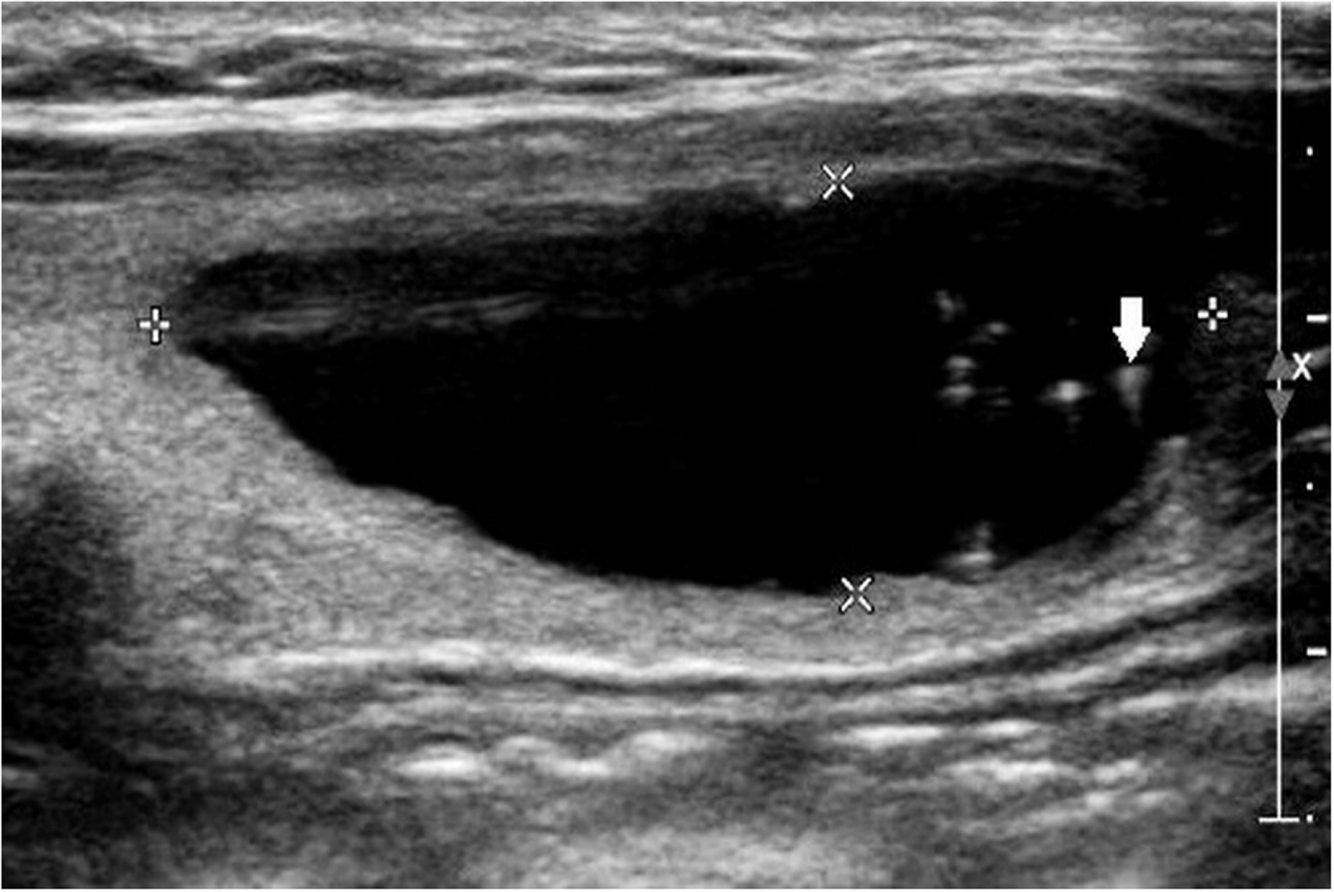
A 46-year-old woman with degenerated multinodular goiter. Longitudinal sonogram shows a 35 mm, smooth, cystic anechoic nodule in the left thyroid lobe. Echogenic foci with comet-tail artifact freely distributed (solid arrow) (Type 1). The patient underwent surgery due to contralateral thyroid malignancy.
